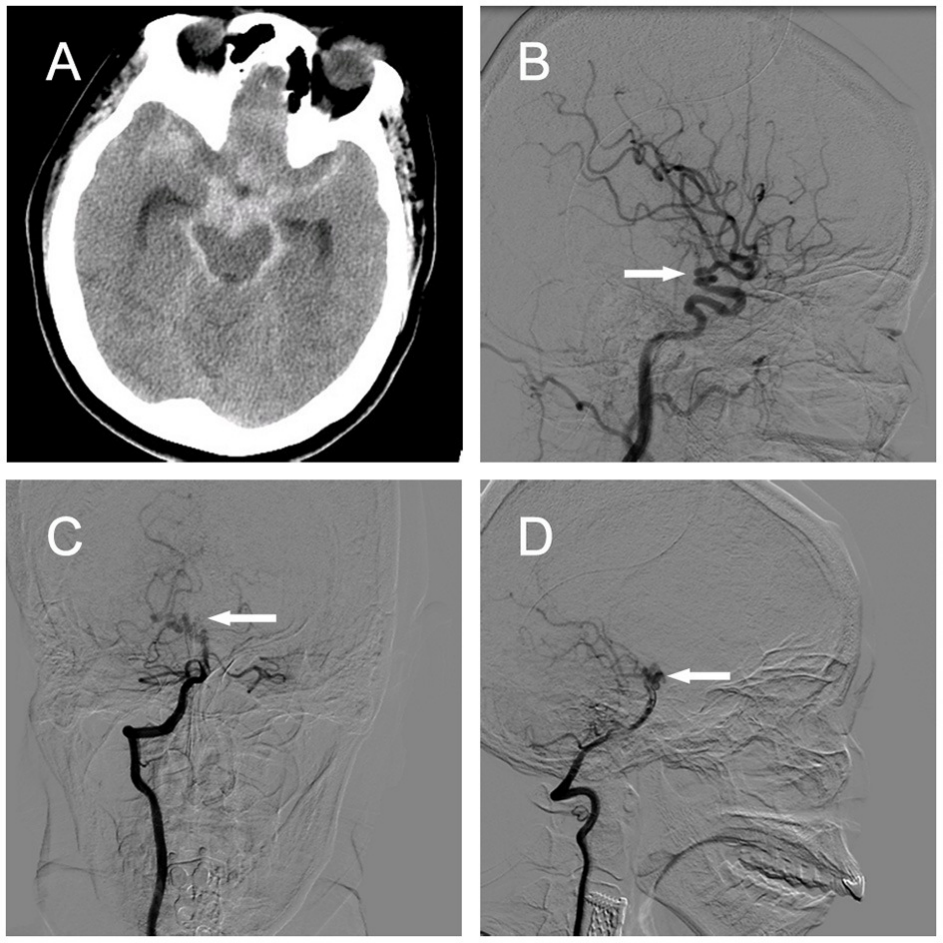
Case 2. (A) Head CT revealing SAH in the suprasellar cistern, ambient cistern, and left sylvian fissure. [(B), arrow] Right ICA angiograms showing a coiled and tortuous vessel of the right supraclinoid ICA in the lateral view. Right vertebral artery angiograms demonstrating a coiled and tortuous right P1 segment in (C) anteroposterior and [(D), arrow] lateral views. ICA, internal carotid artery; CT, computed tomography.
